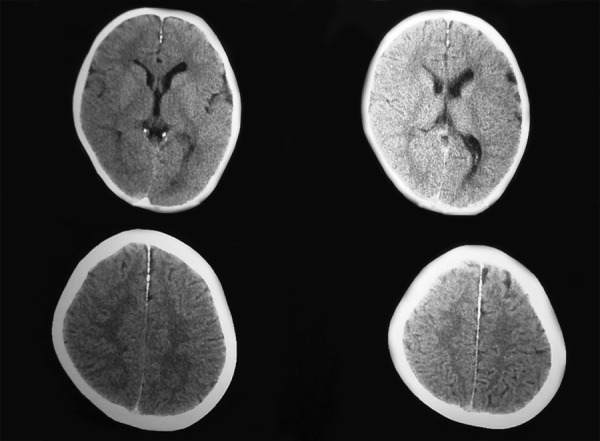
CT of mid face with cyst and nasal polyp
